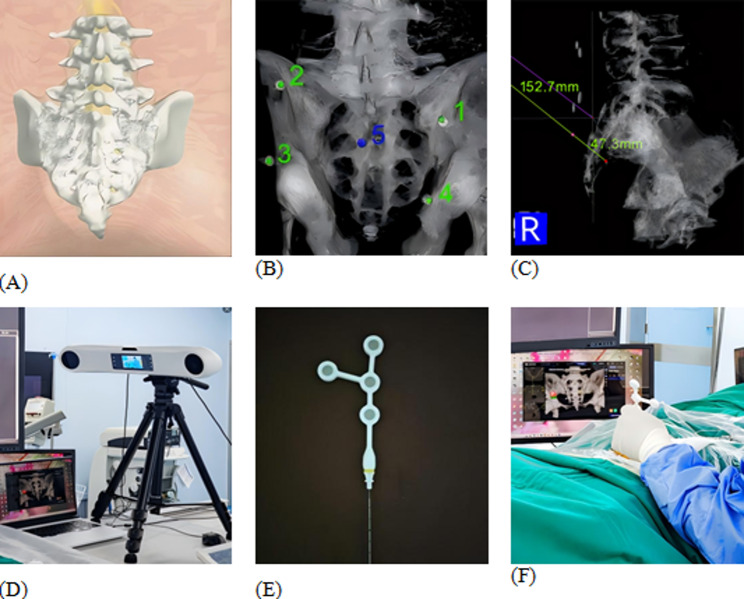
Optical navigation system (ONS) setup and multimodal image fusion. A Multimodal fusion of CT and MRI images. B Placement of five adhesive skin markers. C Manual trajectory planning. D The ONS cart positioned adjacent to the patient. E Needle cap with optical reflective spheres attached to the sacral nerve puncture needle. F Real-time needle puncture guided by ONS
